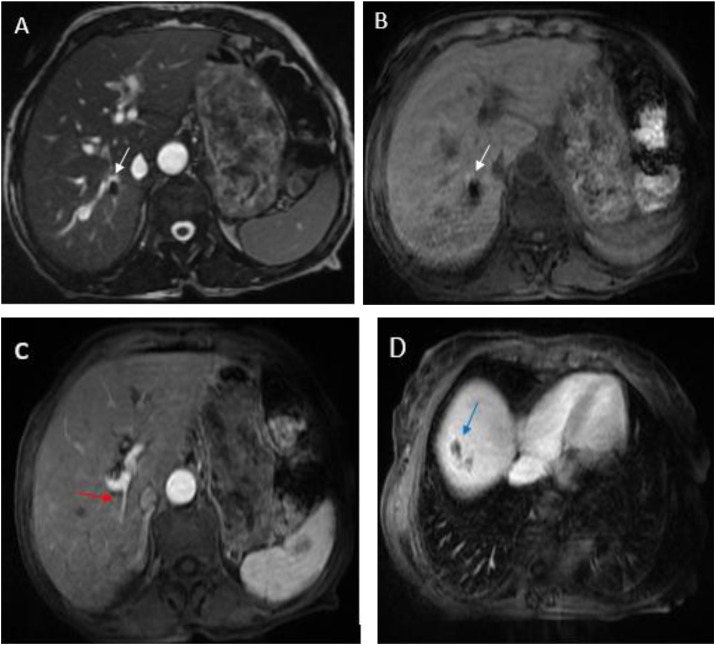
FIESTA and T1-weighted axial sequences, demonstrating the embolization material as T1 and T2 hypointense signal (white arrow) (A and B) with a regular appearance of the hepatic artery wall (red arrow) (C). There is also a significant reduction in the hepatic fluid collection, corresponding to a biloma (blue arrow)(D).
